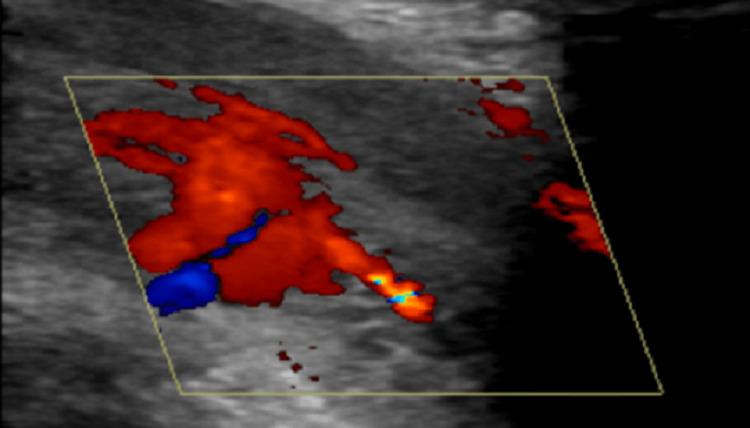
Color Doppler ultrasound image from case 1 demonstrating an arteriovenous fistula with an adjacent hematoma in the right upper cavernosa
